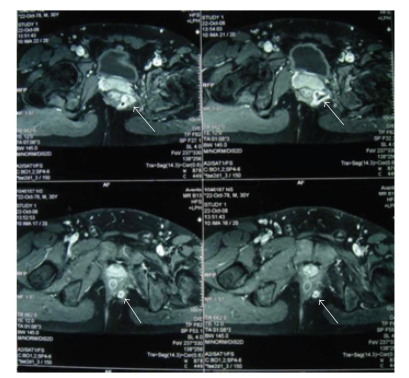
The pelvic MRI of the patient demonstrating the suprasphincteric fistula in ano with abscess formation (arrows).
